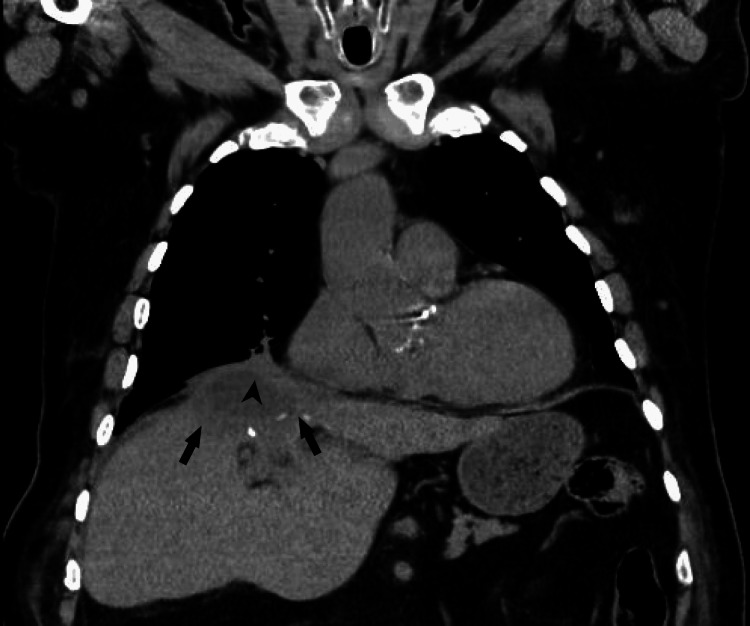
CT scan showing resolution of the hepatic abscess, with only residual scarring remaining (indicated by arrows), and complete cicatrization of the diaphragm (indicated by the arrowhead).
